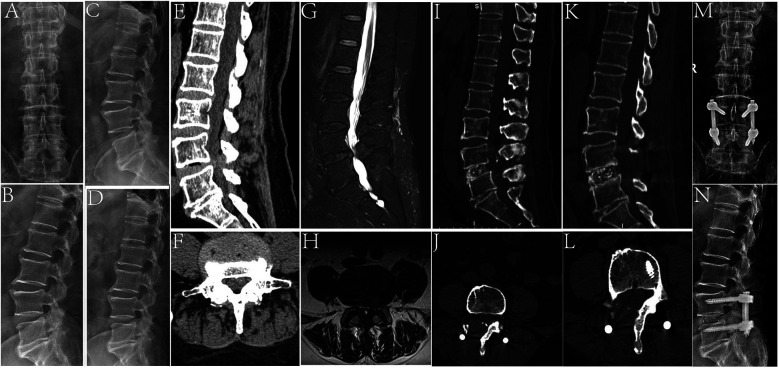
A 76-year-old male patient who underwent UBE-LIF. (A,B) Preoperative anteroposterior and lateral x-ray images. (C,D) Preoperative lumbar imaging in the dynamic position. (E,F) Preoperative lumbar MRI T2 images in the sagittal and transverse planes showed L4/5 spinal stenosis. (G,H) Preoperative lumbar CT in the sagittal and transverse positions. (I,J) Postoperative lumbar CT in the sagittal and transverse positions showed complete decompression of the spinal canal. (K,L) The last follow-up of lumbar CT in the sagittal and transverse positions showed no significant abnormality in the surgical area and the vertebral body had fused. (M,N) Postoperative x-ray showed that the screw was fixed in place.
